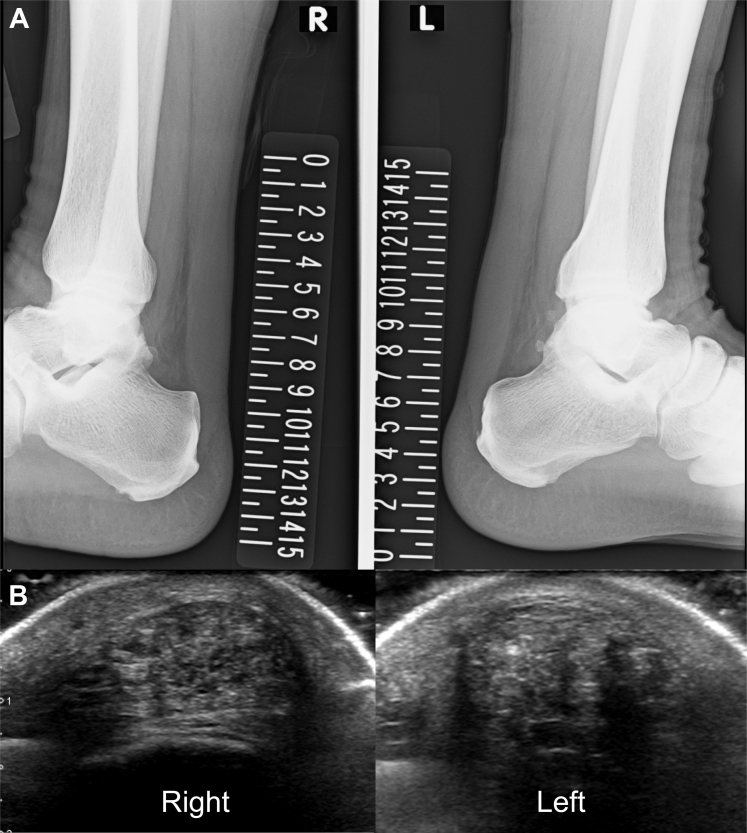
Structural Imaging of Achilles Tendons in the 71-Year-Old Father (A) Lateral ankle radiography showing bilateral Achilles tendon thickening with microcalcifications. (B) Ultrasonography demonstrating moderate thickening (right: 8.3 mm, left: 9.5 mm) and heterogeneous echotexture.
